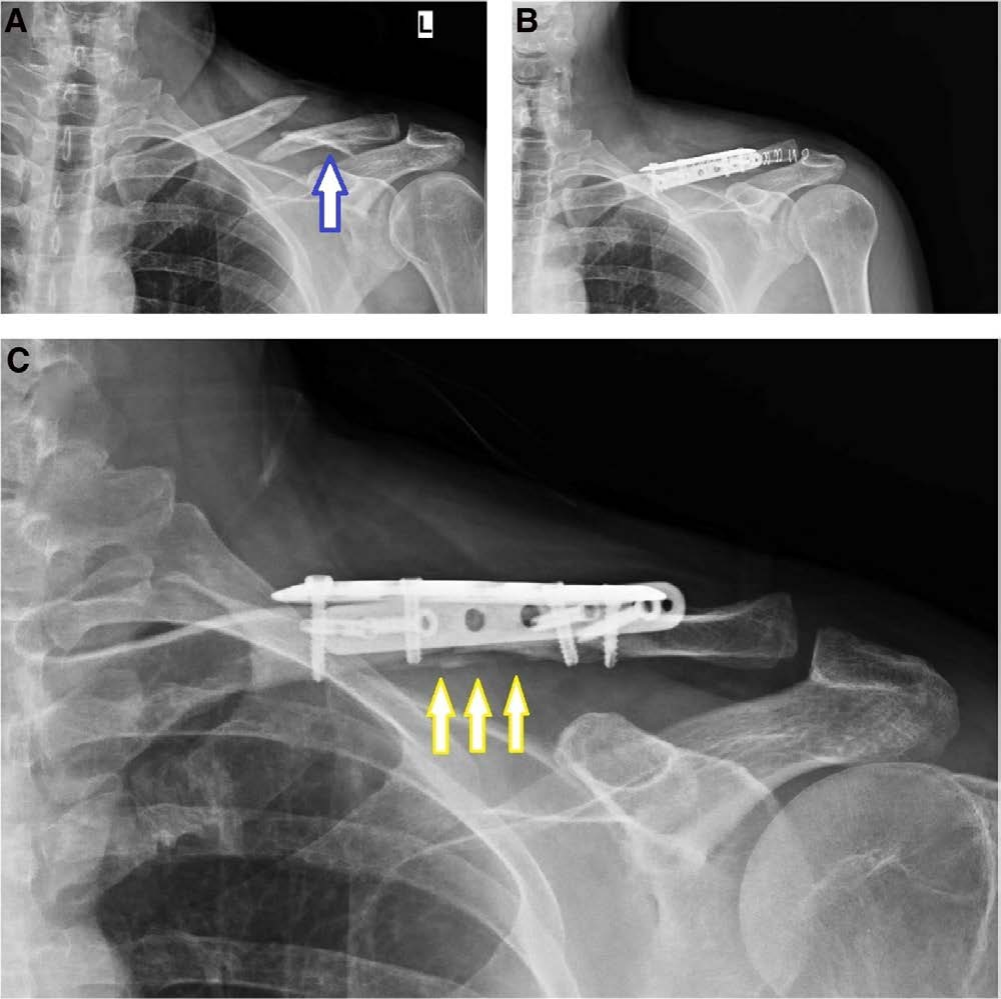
(A) Preoperative X-ray of the 45 years old, male patient, multi-fragmented clavicle fracture; blue arrow shows comminuted fracture fragment. (B) First postoperative X-ray of the patient, fracture fixation was achieved with plates placed on the superior and anterior of the clavicle. (C) Patient’s X-ray at 3rd month postoperatively; yellow arrow shows the callus bridge at the fracture line.
